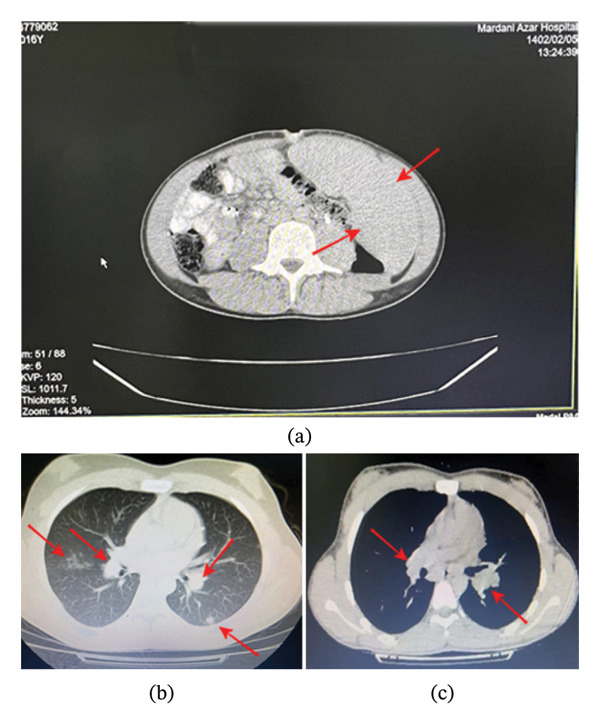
(a) Abdominal CT scan: massive splenomegaly assigned with red arrows. (b) A chest CT scan shows lymphadenopathy on both sides, along with interstitial involvement, subpleural nodules, and per‐fissure nodules (assigned with arrows). (c) Chest CT: bilateral lymphadenopathy (window view).
